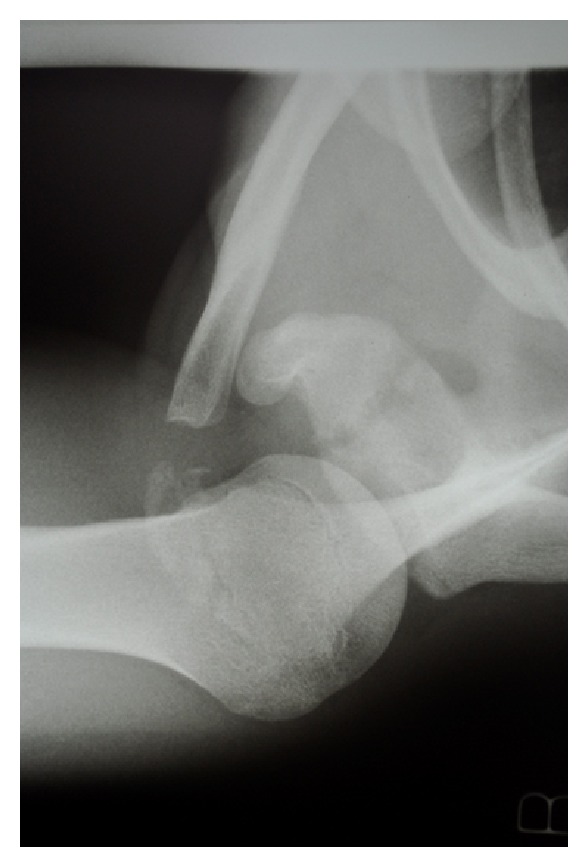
Case A: axillary radiograph at 9 weeks post injury.
